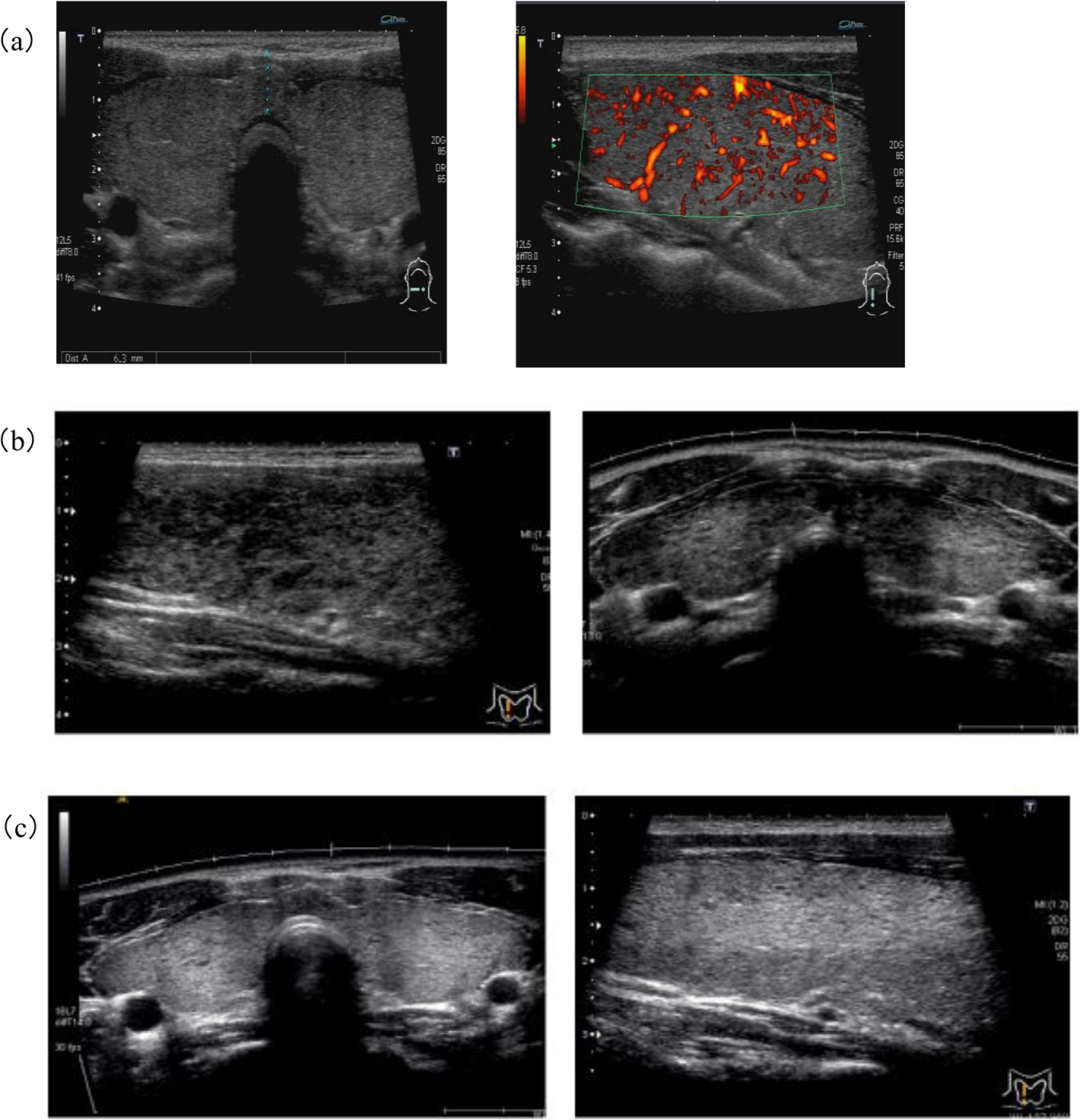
Thyroid ultrasonography in each phase: (a) the SITSH state, while the patient was hospitalized in August 2015; (b) the third thyrotoxicosis episode in January 2018; and (c) recovery from painless thyroiditis in May 2019. (a) Moderate goiter, slightly heterogeneous hypoechogenicity, and moderately rich thyroid blood flow were detected. (b) There were focal hypoechoic areas without tenderness. (c) The hypoechoic area became invisible, and the echogenicity and homogeneity returned to the original condition
